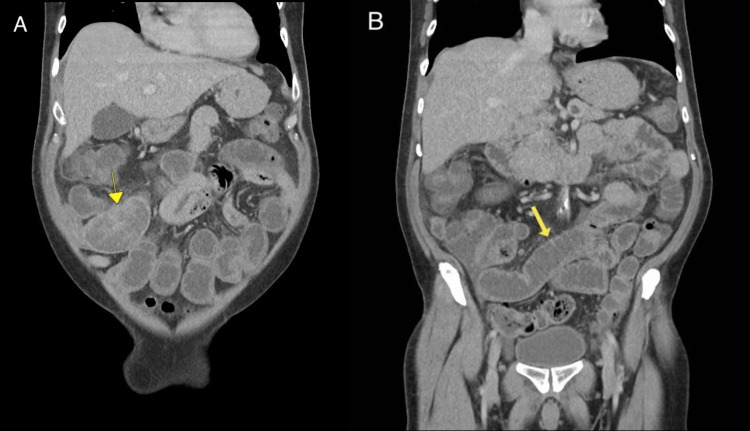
CT of the abdomen: A 2.2 x 4.9 cm mass (yellow arrow) is seen in the distal ileum (A), and the small bowel loops proximal to the mass appear dilated (B). CT - computed tomography
